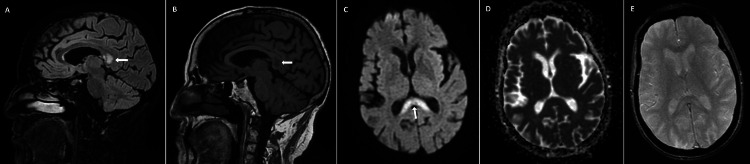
Brain magnetic resonance imaging performed 10 days after metronidazole discontinuation (A) Sagittal T2-fluid-attenuated inversion recovery (FLAIR) image showing a hyperintense lesion involving the splenium of the corpus callosum (white arrow); (B) sagittal T1-weighted image demonstrating hypointensity of the lesion (white arrow); (C) axial diffusion-weighted imaging (DWI) showing hyperintensity of the lesion (white arrow); (D) axial apparent diffusion coefficient (ADC) map showing no evidence of restricted diffusion; (E) axial T2*-weighted gradient-echo (GRE) image demonstrating no susceptibility changes
